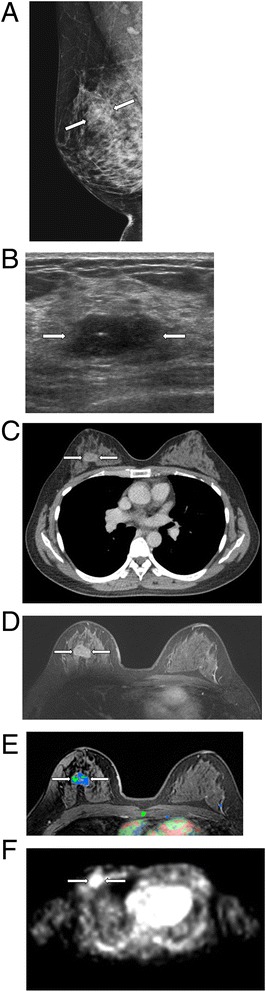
How to measure the tumor extent. The longest diameter of a breast tumor was recorded using each modality; MMG (A), US (B), CT (C), MRI without CAD (D), MRI with CAD (E), and FDG-PET (F). For measurement of tumor extent with CAD, an enhancing tumor above the threshold was selected with the color overlay map, and then CAD-generated maximum tumor size was recorded.
